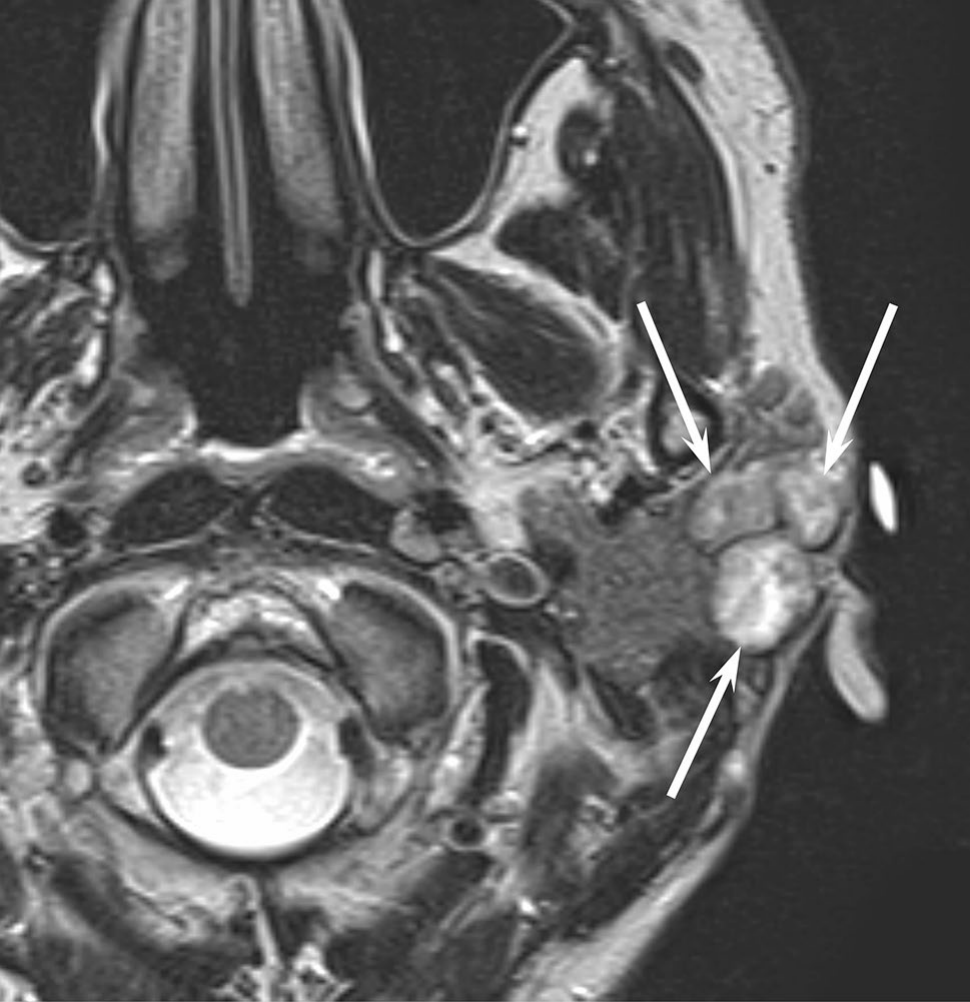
A 34-year-old woman with myoepithelioma of the left parotid gland. T2-weighted image shows a multinodular lesion with lobulated margin and intratumoral septa (arrows)
